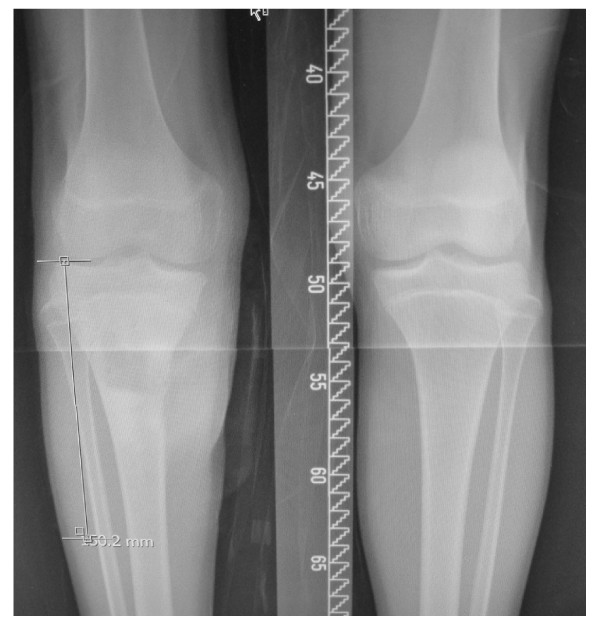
Plain radiograph showing a mixed sclerosis and lytic lesion over the right upper tibia and break in the medial cortex.
